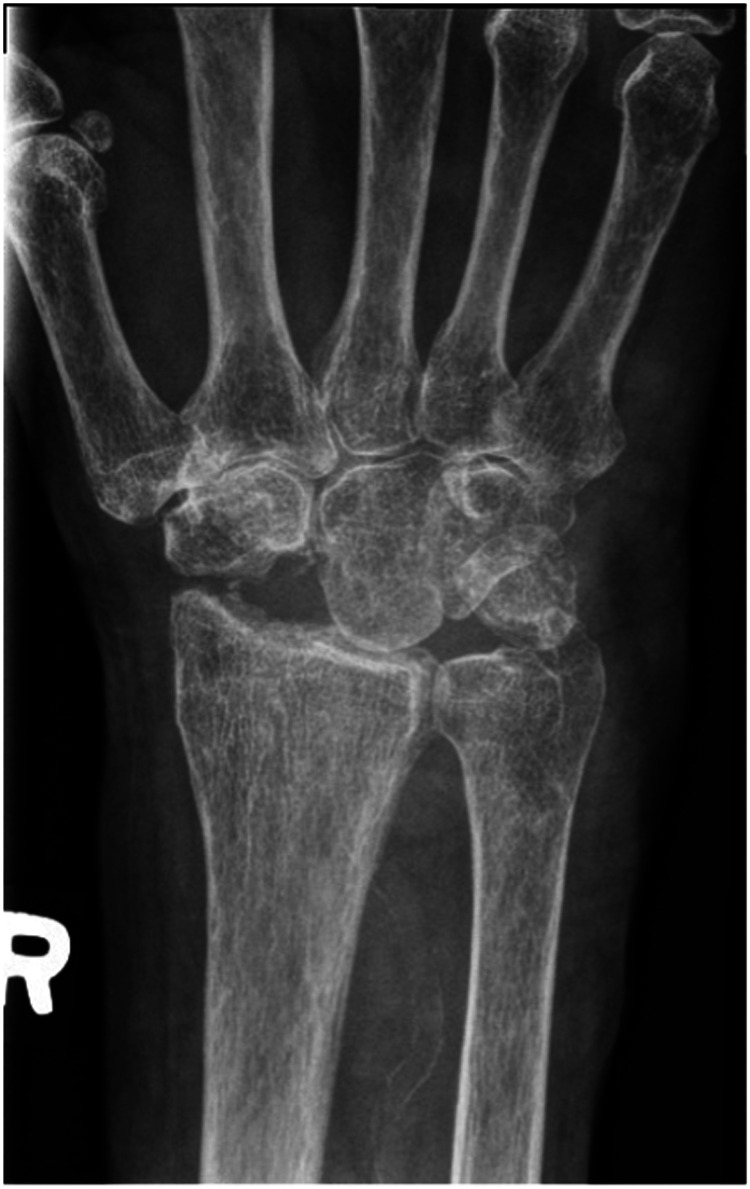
Postoperative right hand plain film posterior to anterior view x-ray.
